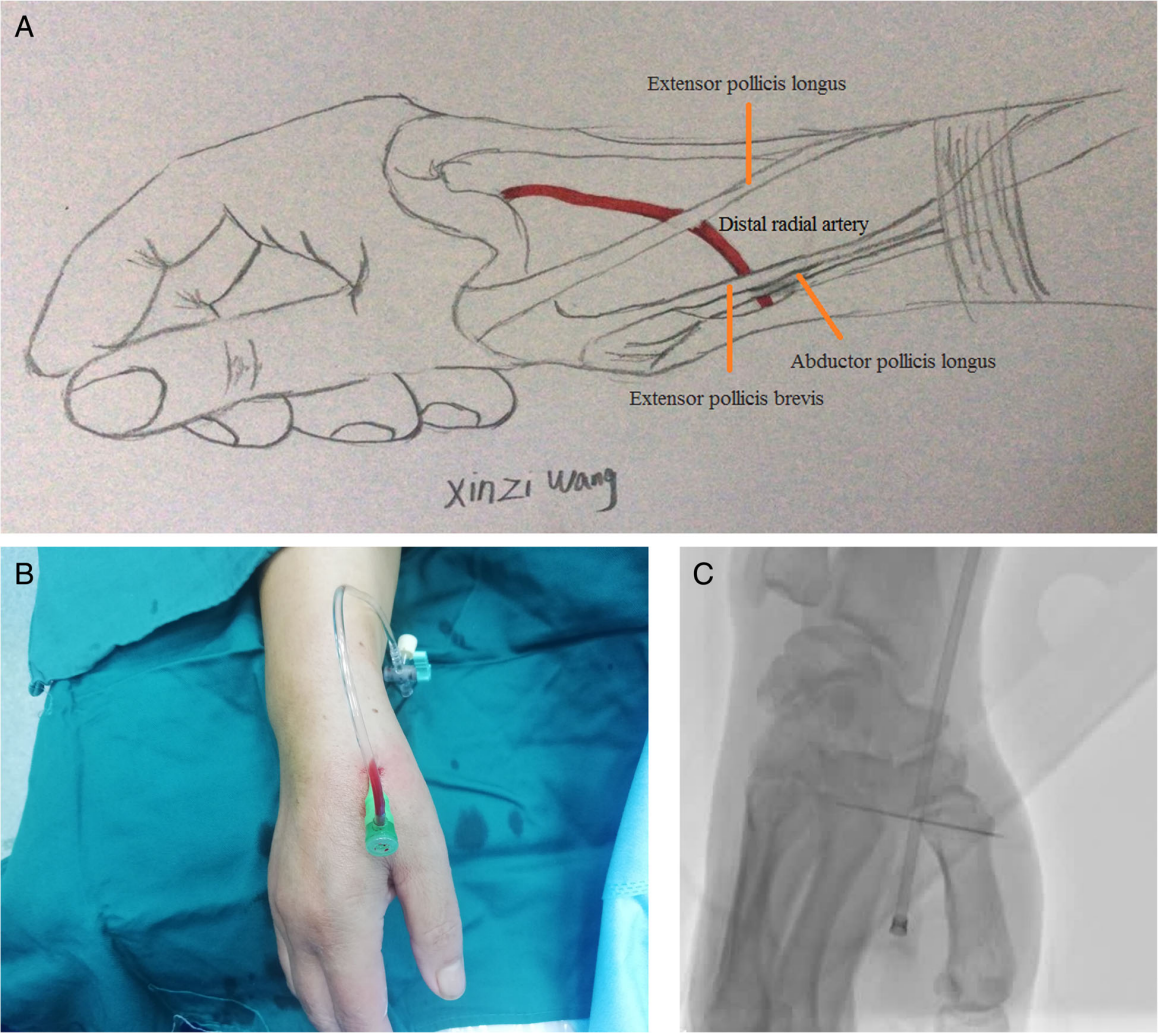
Anatomy of DRA (a. Sketch of DRA; b. 6Fr Sheath implanted into DRA; c. Location of the puncture site under X-ray)
